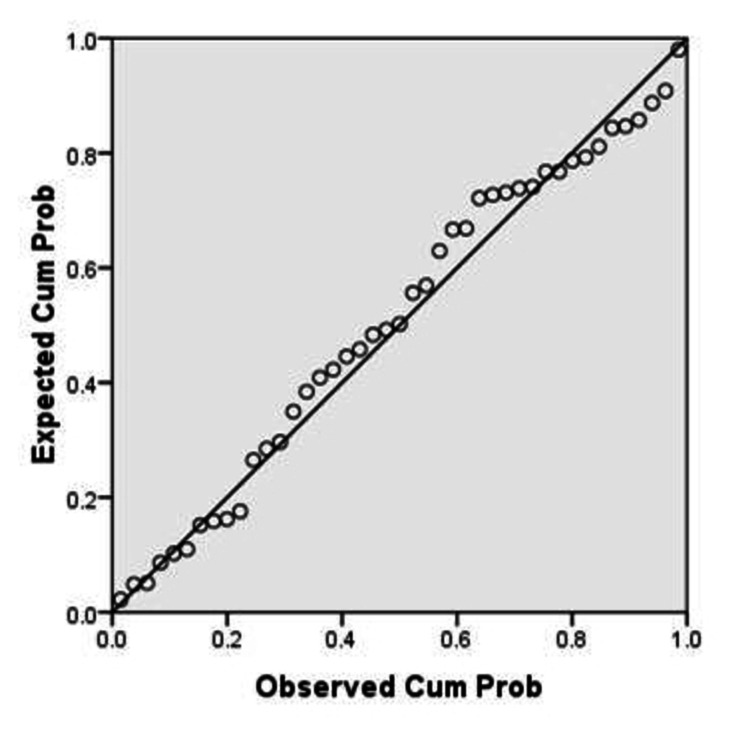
P-P plot Dependent variable: %BF US %BF, body fat percentage; US, ultrasound
